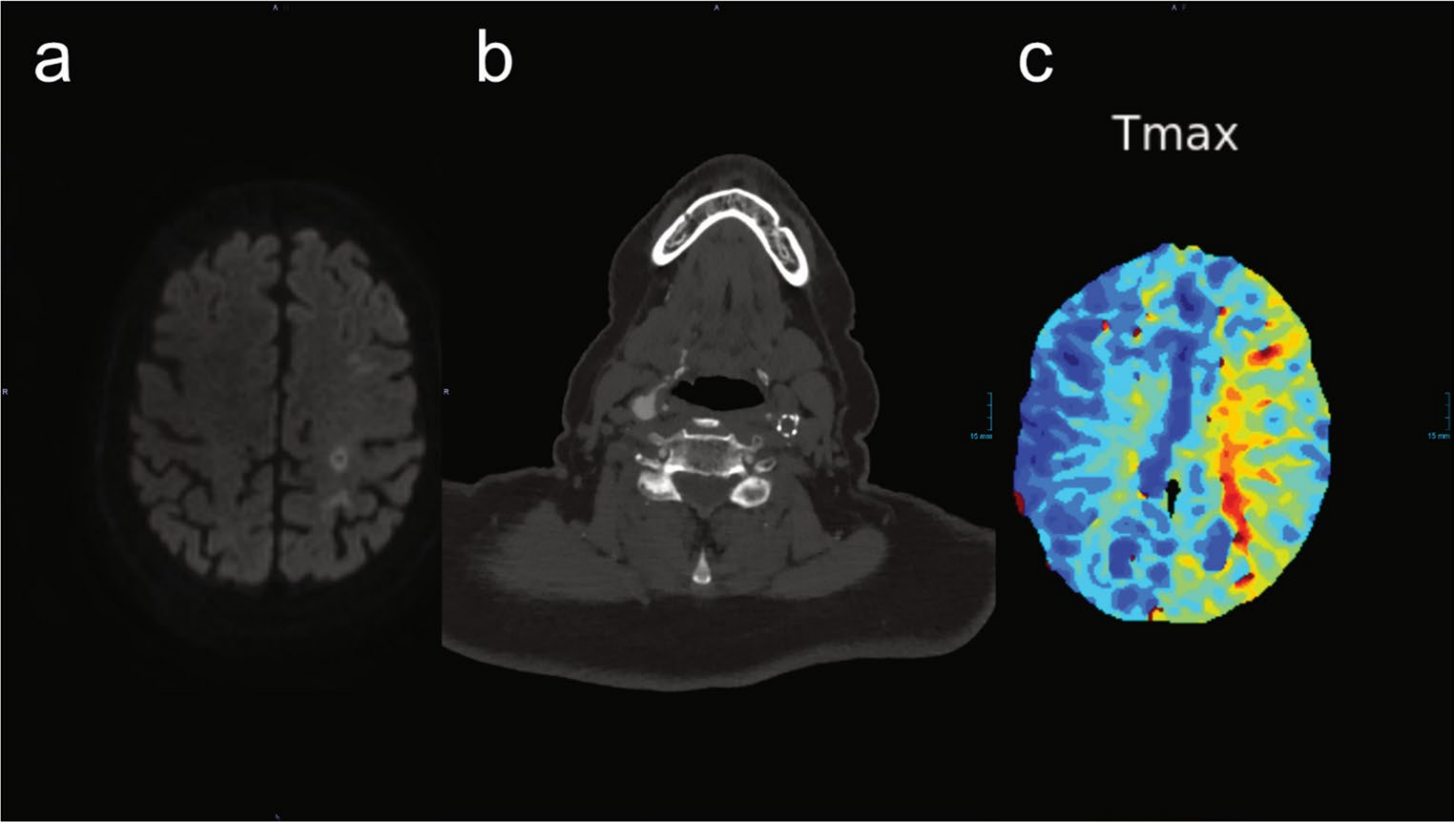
MRI and CTA on admission showing (a) acute left watershed infarctions and (b) stent occlusion with (c) remarkable perfusion impairment in CT-P
